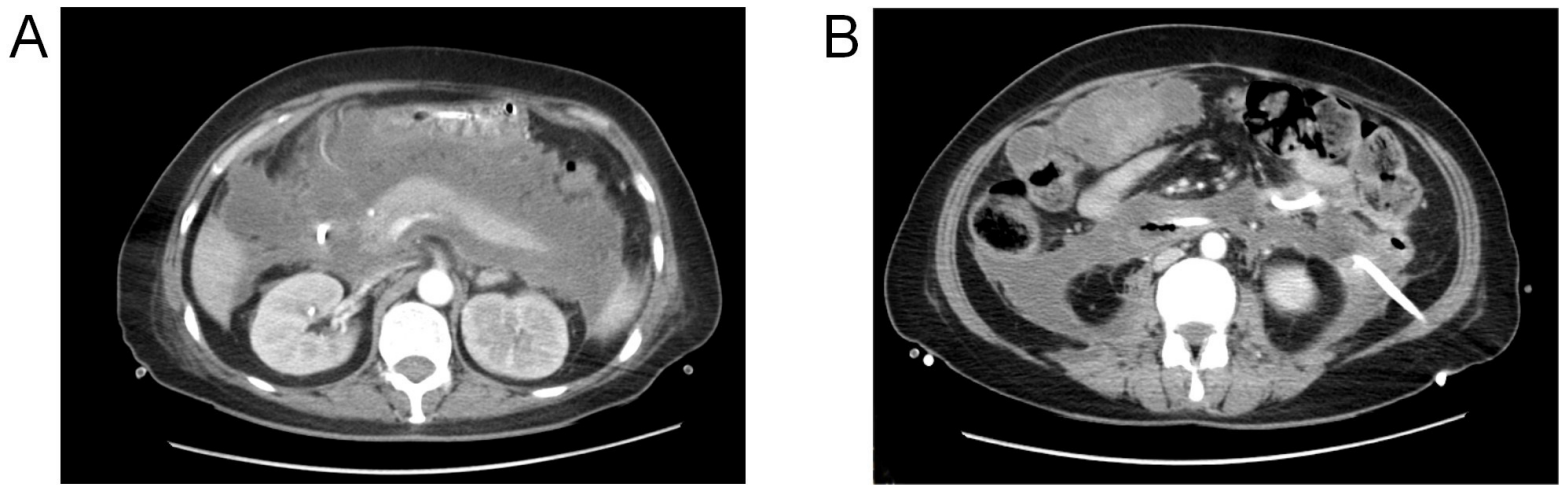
(A) Peri-pancreatic necrosis demonstrated on contrast-enhanced computed tomography (CECT); (B) retroperitoneal drainage catheter (12Fr).
